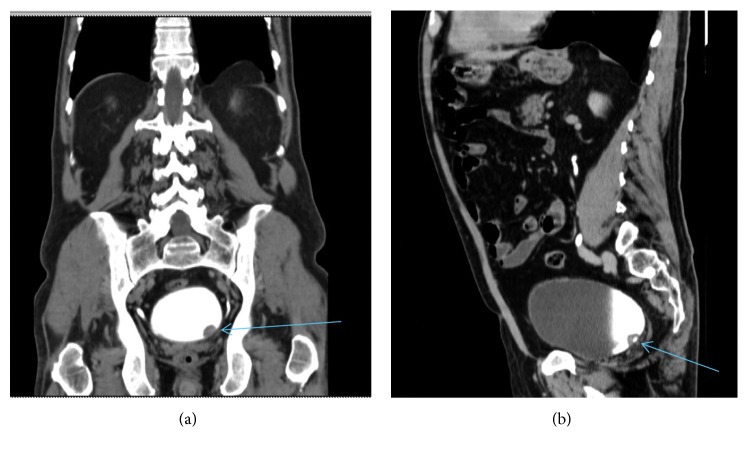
Coronal sections Uro-CT scan with contrast showing filling defect (arrows).
